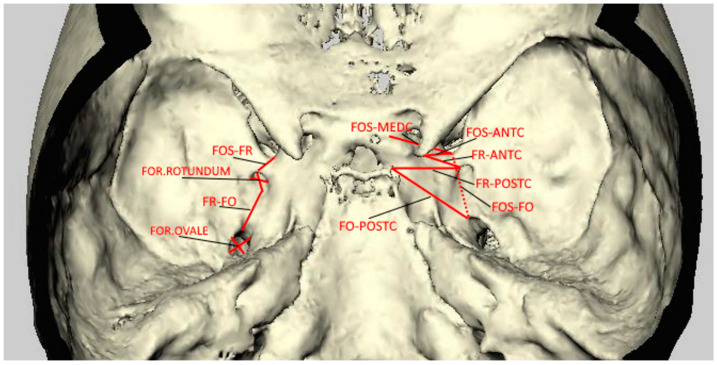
3D representation of the CT image showing all intracranial measurements.
